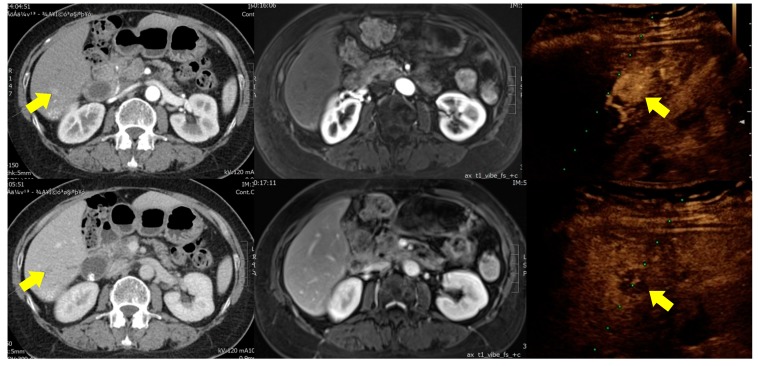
A 65-year-old woman with chronic hepatitis B infection was shown to have an S6 liver tumor. CT scans revealed trace enhancement in the arterial phase (left upper part) and mild wash-out in the portal phase (left lower part). MRI exam failed to detect the tumor (middle part). CEUS analysis showed strong enhancement in the arterial phase (right upper part) and typical wash-out in the Kupffer phase (right lower part). Biopsy confirmed a diagnosis of hepatocellular carcinoma.
